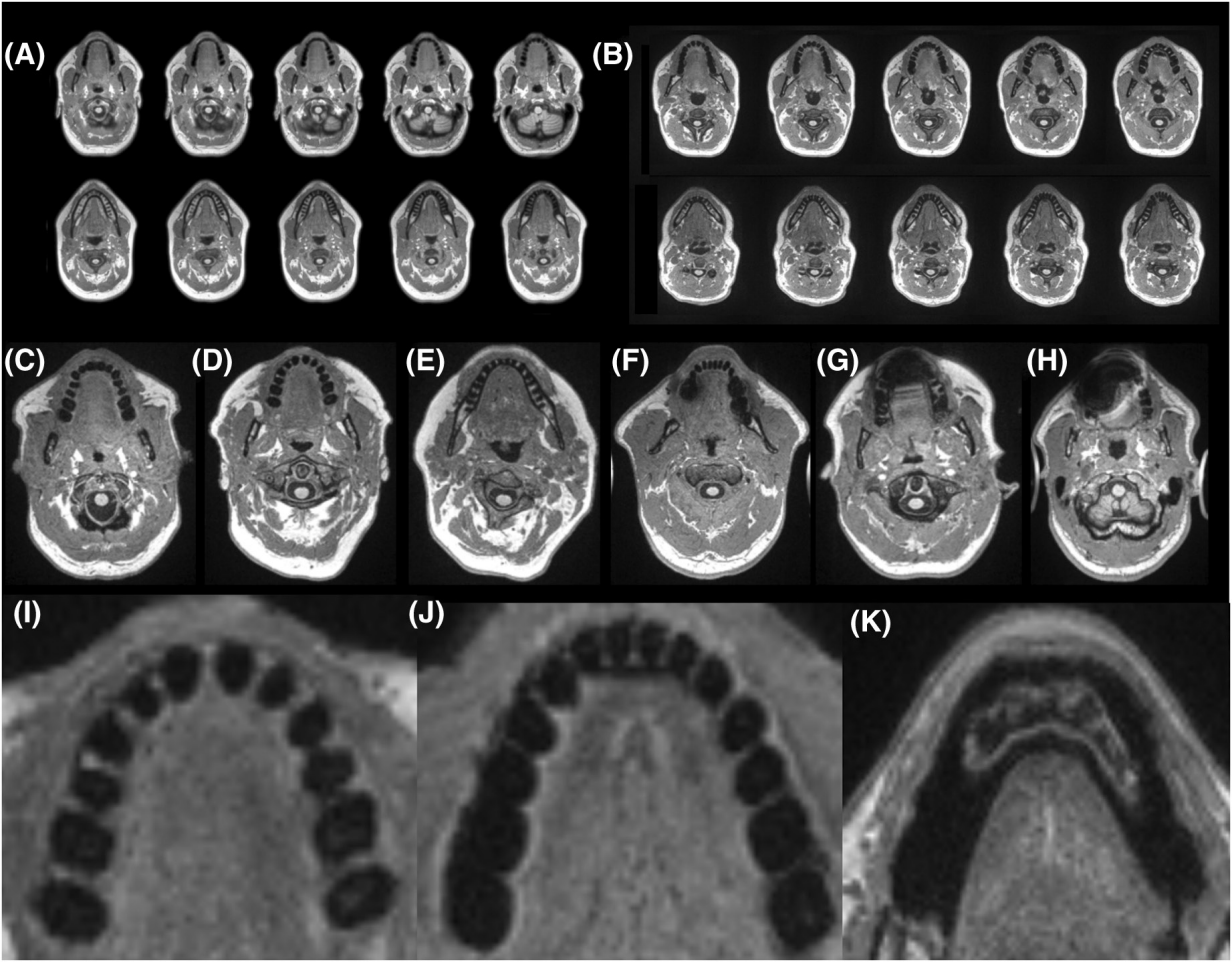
Teeth were manually counted based on visual inspection of axial images extracted from T1‐weighted MRI scans. (A, B) Sequential axial slices showing the upper (top row) and lower (bottom row) jaw for two individual participants without any MRI distortion and for whom calculation of upper and lower teeth was completed. (C) Example key axial image from a participant with 14 enumerable upper teeth (D) Example key axial image from a participant with 12 enumerable upper teeth. (E) Example key axial image from a participant with 16 enumerable lower teeth. (F) Minimal bilateral distortion caused by implants in the left and right lower teeth. (G) Significant midline distortion caused by a retainer affecting upper and lower jaw. (H) Significant distortion in the area of the upper teeth caused by dental bridge. (I–K) Visualization software (MRIcroGL12, https://github.com/rordenlab/MRIcroGL) allowed raters to zoom in on axial slices while scrolling in the superior/inferior direction, thus making tooth enumeration easier.
